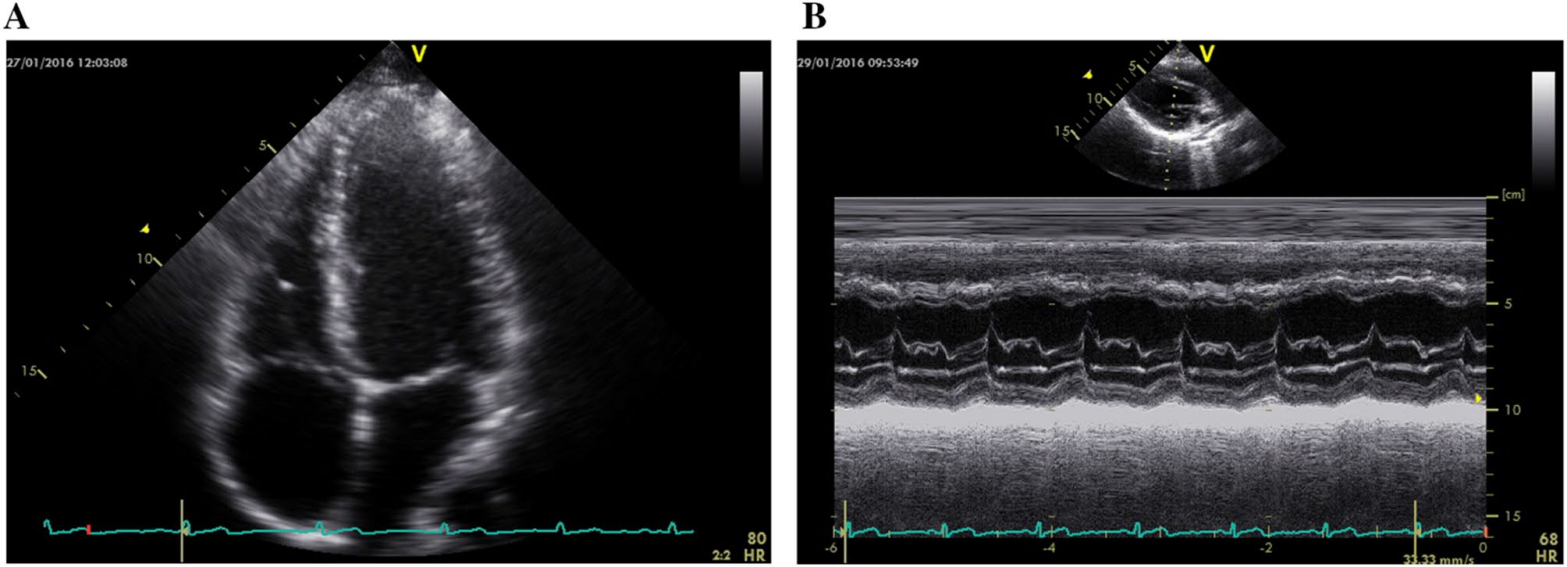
a Experimental frame extracted from a captured cineloop of the apical 4 chamber view. b Experimental image capture of the 4-chamber parasternal long axis view observed during M-mode recording
